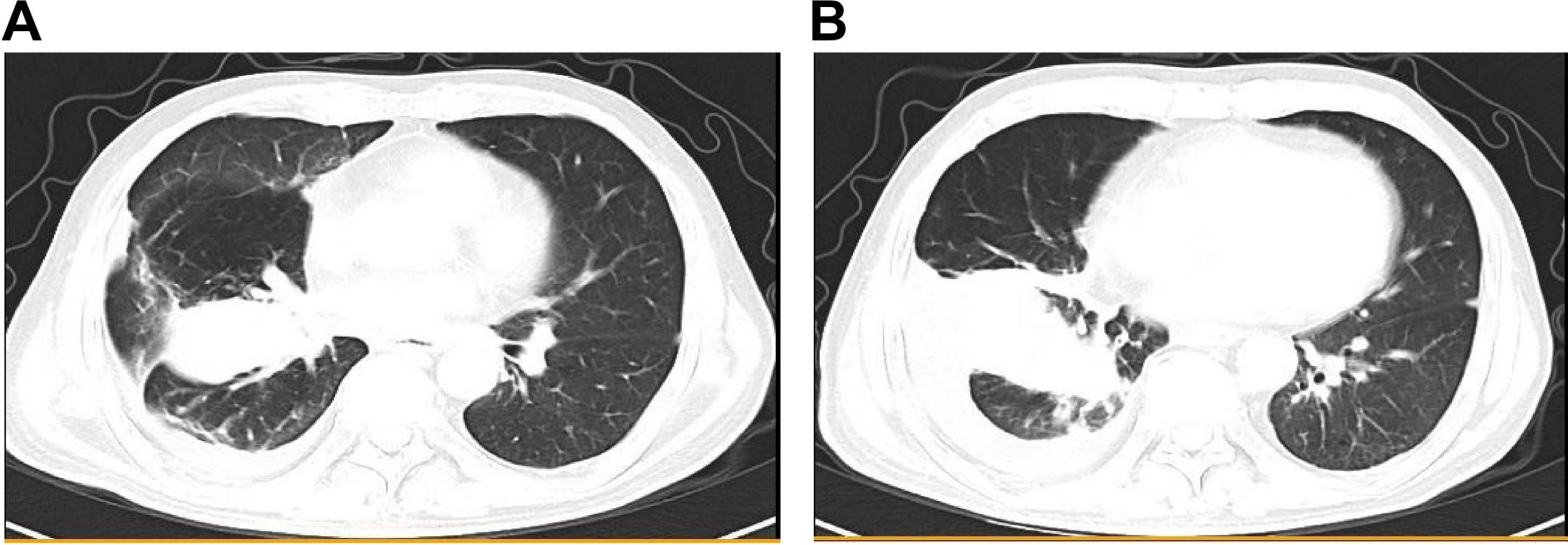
Chest CT on 16 October 2022. The space‐occupying lesion in the right lower lobe has decreased in size compared with 4 May 2020, while the range of obstructive pneumonia in the right lower lobe has increased compared with before. Remaining bilateral lung inflammation has improved since the previous assessment, and the pleural effusion on the right side has decreased in extent.
